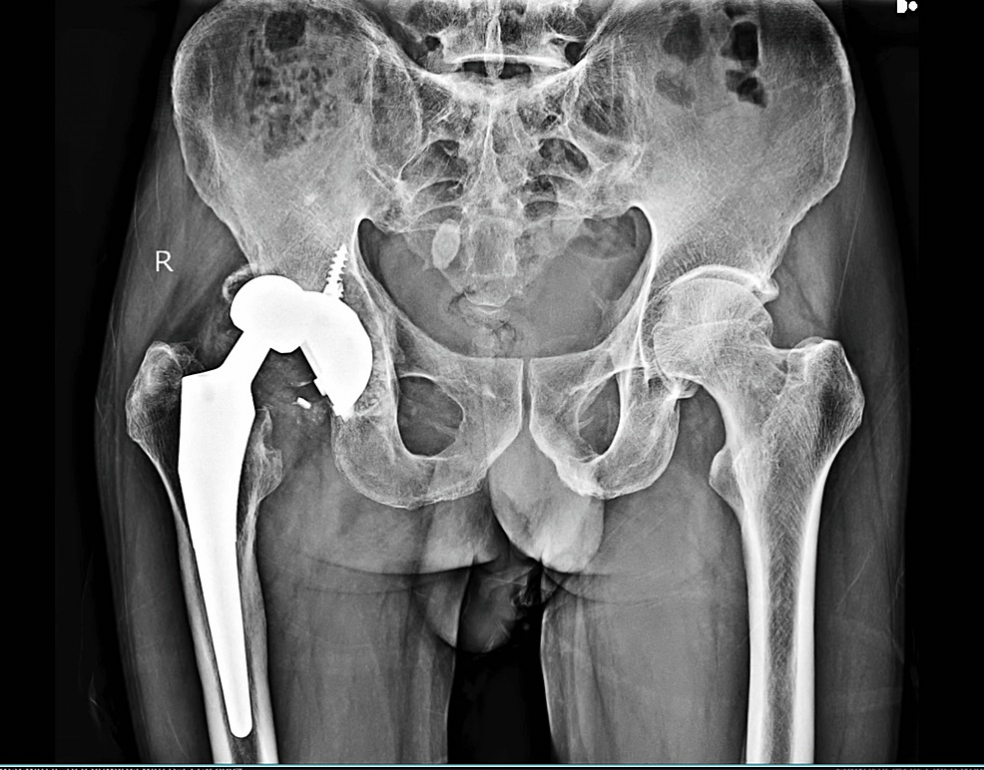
Pre-operative X-ray of the pelvis with both hips (anteroposterior view)
